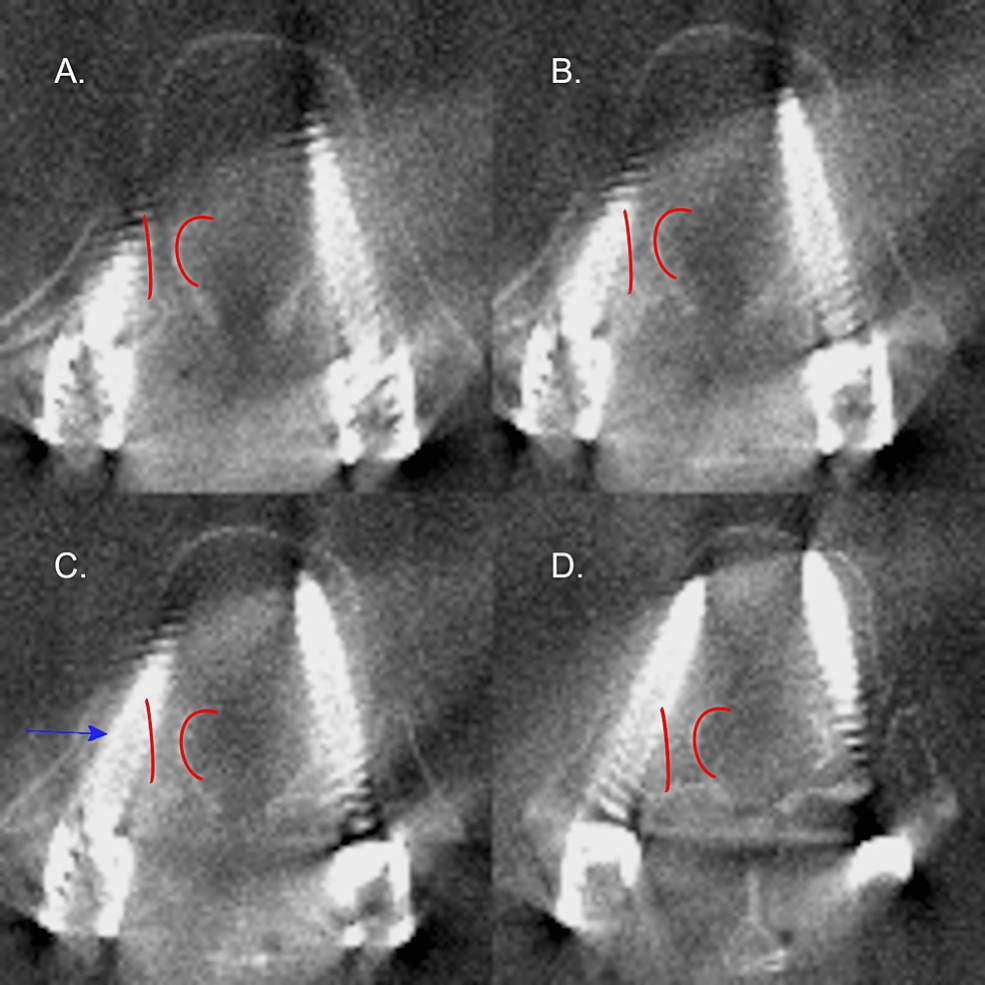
Axial CT radiographs of the right T12 pedicle screw on patient 4. Axial CT radiographs demonstrating the right pedicle screw placement on patient 4 at T12 with an in-out-in (IOI) trajectory. Screw placement progresses from A to D. The pedicle of interest is outlined in red. The blue arrow denotes the largest deviation of the screw. Please note all CT images are in radiographic orientation.
